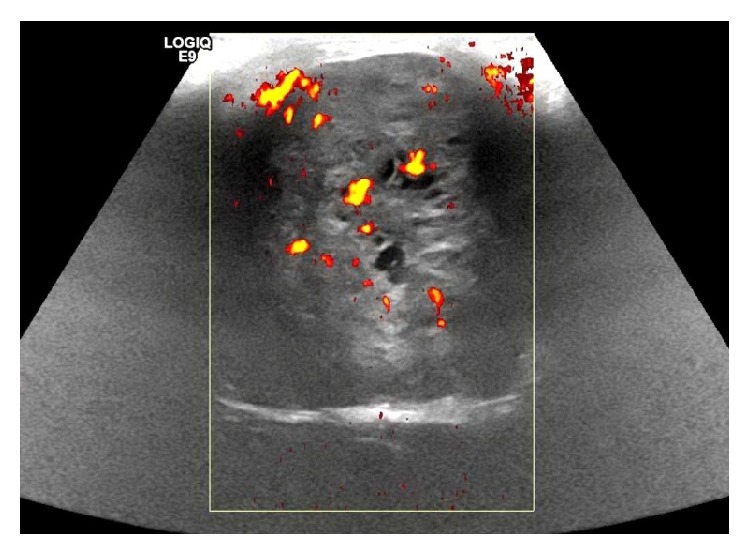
Ultrasound of groin showing complex vascular mass.
